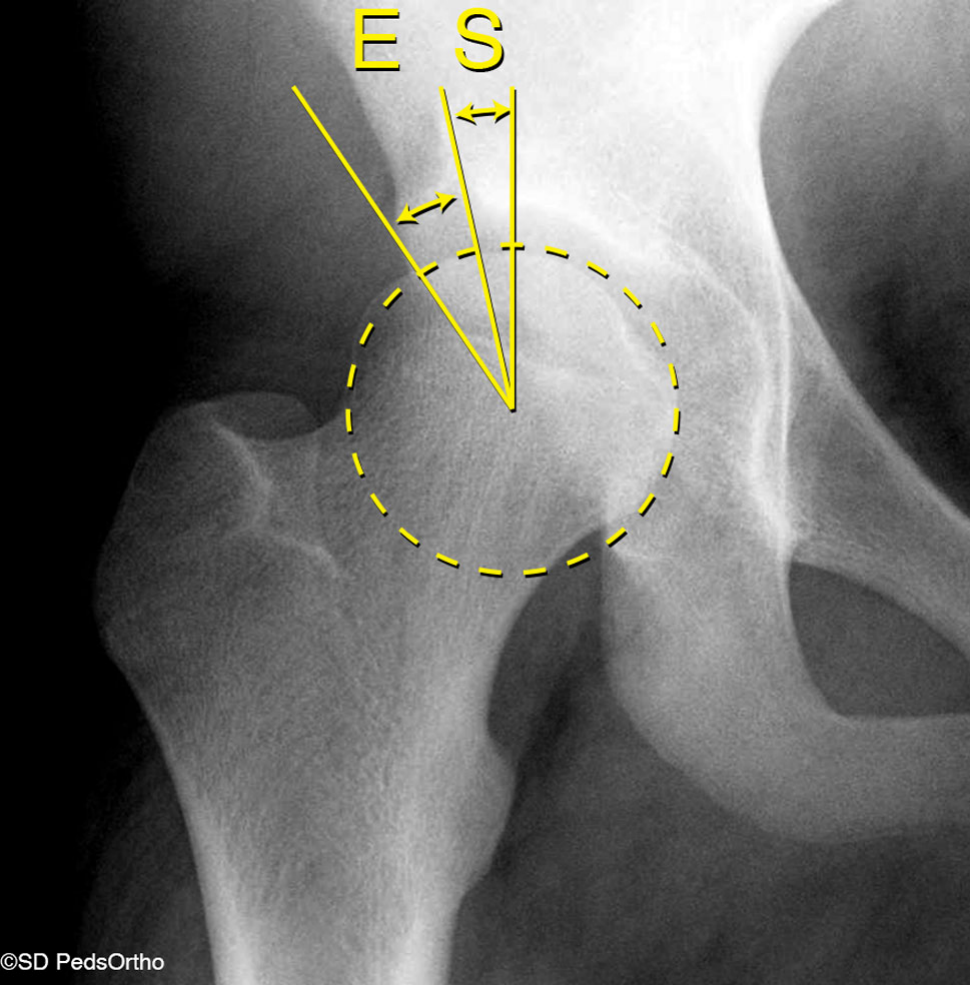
Anteroposterior X-ray of the right hip indicating the method of measurement for the lateral center edge angle measured to the edge of the acetabulum, as described by Wiberg (*E*) and to the edge of the sourcil (*S*)
